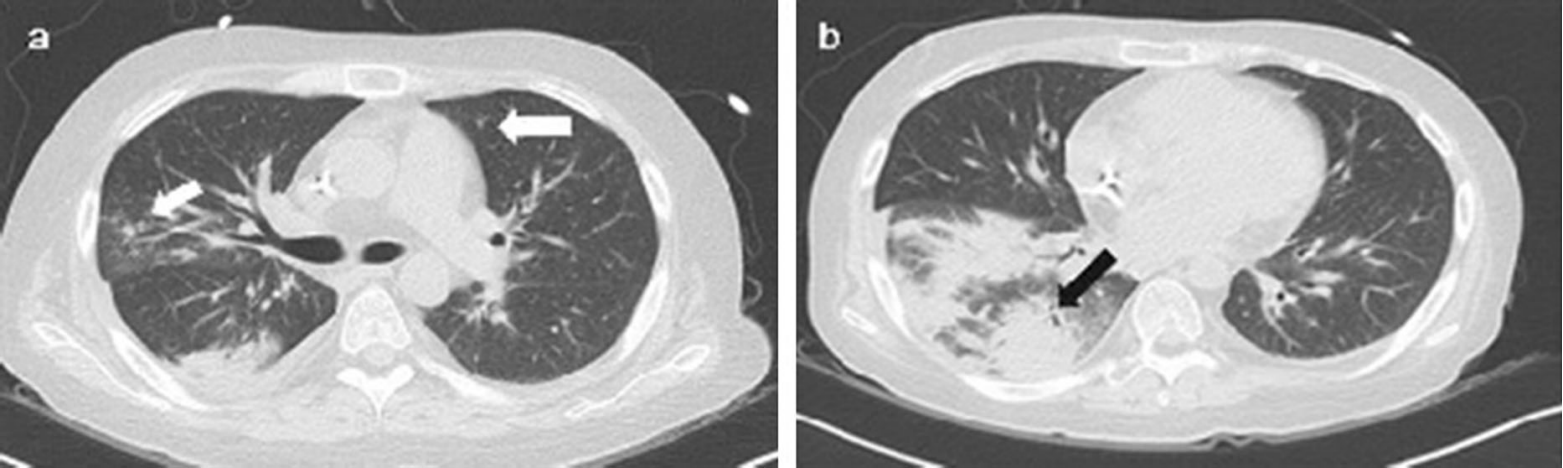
Low-dose chest computed tomography. a Ill-defined centrilobular nodules in both lungs (white arrows). b Peribronchial consolidation that accompanied by ground-glass opacities
